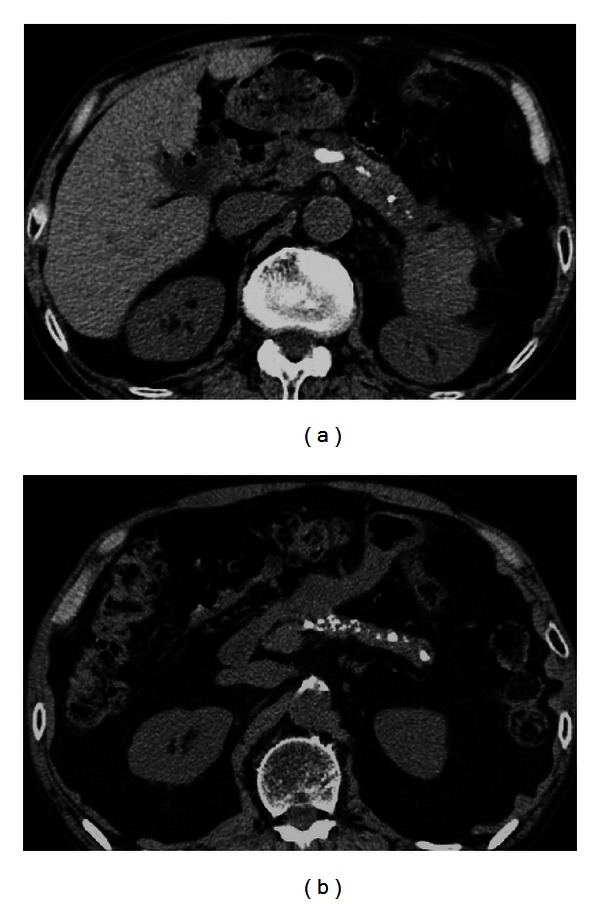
CT of AIP showing definite imaging findings. (a) Stones in pancreatic ducts. (b) Multiple or numerous calcifications distributed throughout the entire pancreas.
